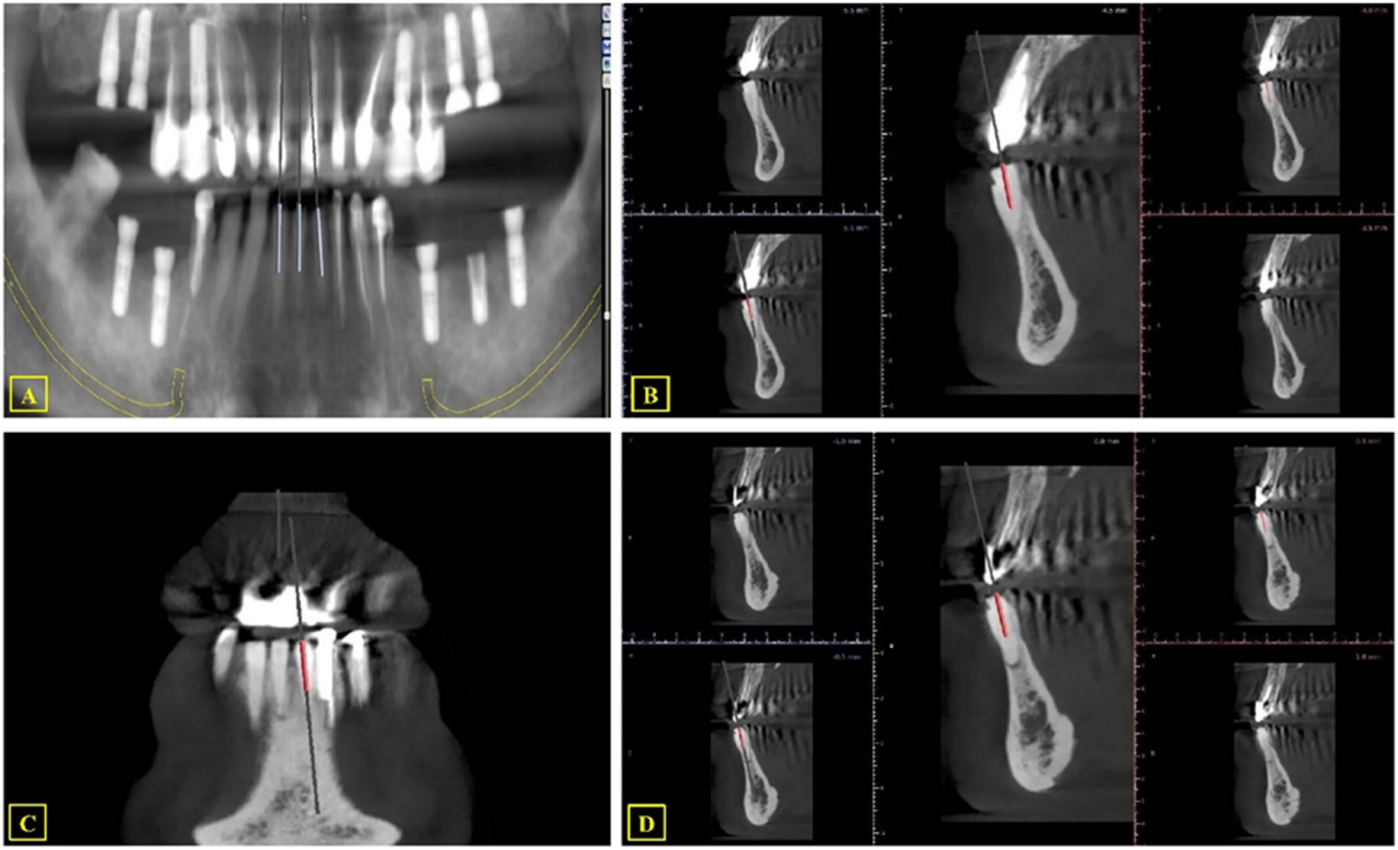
The drill guide path design was based on a three‐dimensional assessment of CBCT slices. (A–D).
